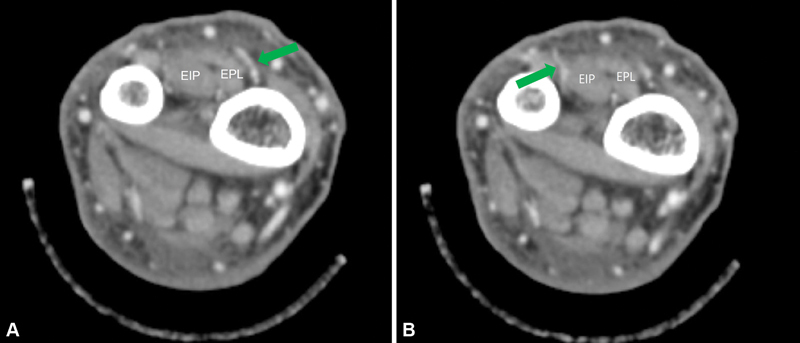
Preoperative upper extremity CT angiography. (
